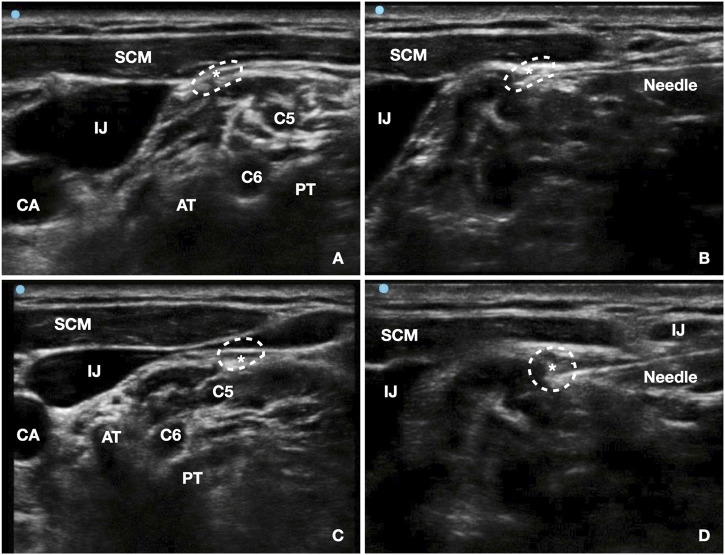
Ultrasound-guided bilateral phrenic nerve pulsed radiofrequency (PNRF) treatment. (A) Right-side PNRF localization view; (B) Right-side in-plane needle placement view; (C) Left-side PNRF localization view; (D) Left-side in-plane needle placement view. AT: anterior tubercle; PT: posterior tubercle; CA: carotid artery; IJ: internal jugular vein; SCM: sternocleidomastoid muscle; C5/C6: corresponding cervical nerve roots; *phrenic nerve indicated by white dotted circle.
